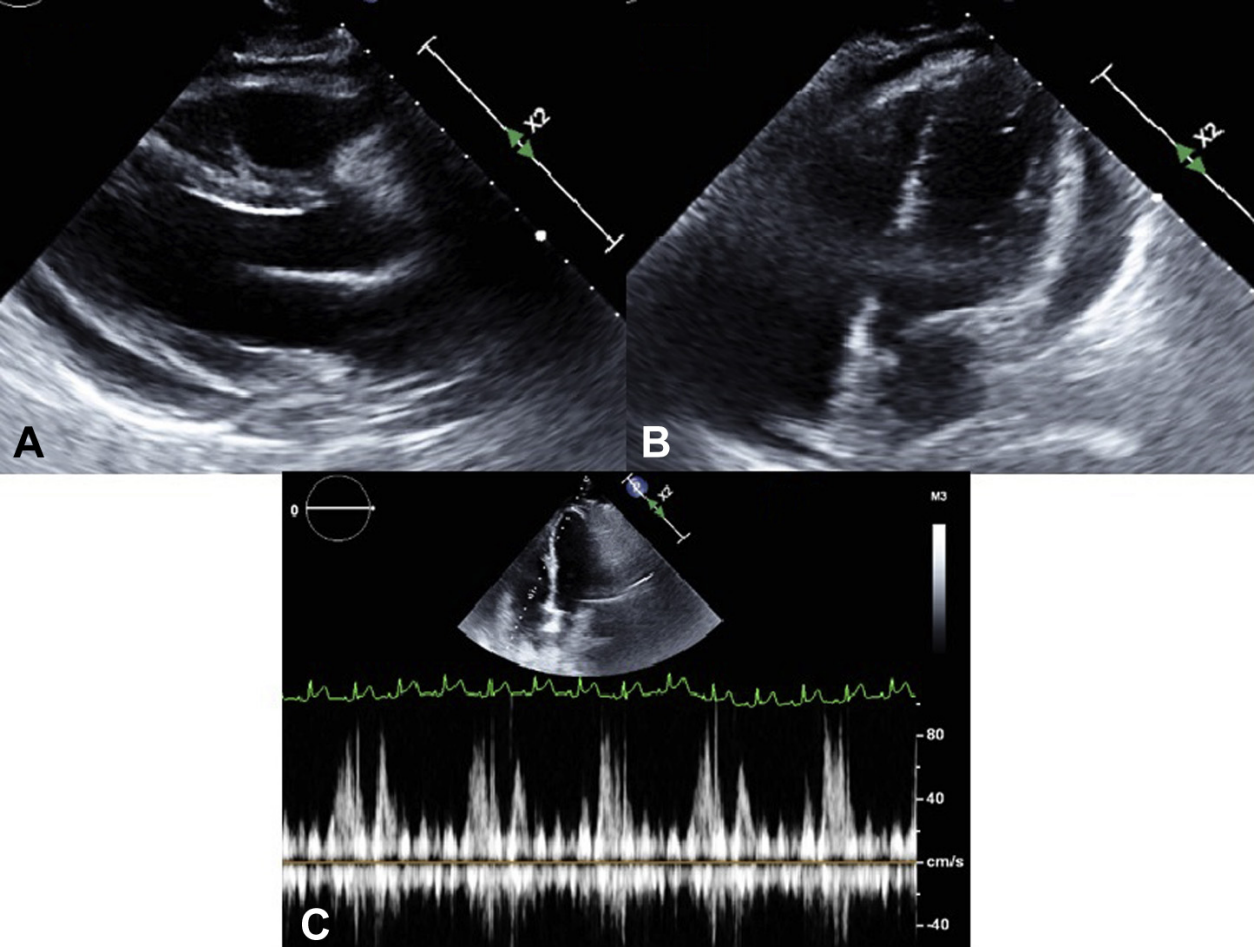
Echocardiogram on day 3. (A) Parasternal long-axis view revealed moderate-size circumferential pericardial effusion. (B) Apical 4-chamber view revealed moderate-size circumferential pericardial effusion. (C) Pulse-wave Doppler across the tricuspid valve revealed significant respiratory inflow variation. Every third cardiac cycle is associated with absent tricuspid inflow, presumably during expiration, with the patient breathing at a rate of 30/min, consistent with tamponade.
